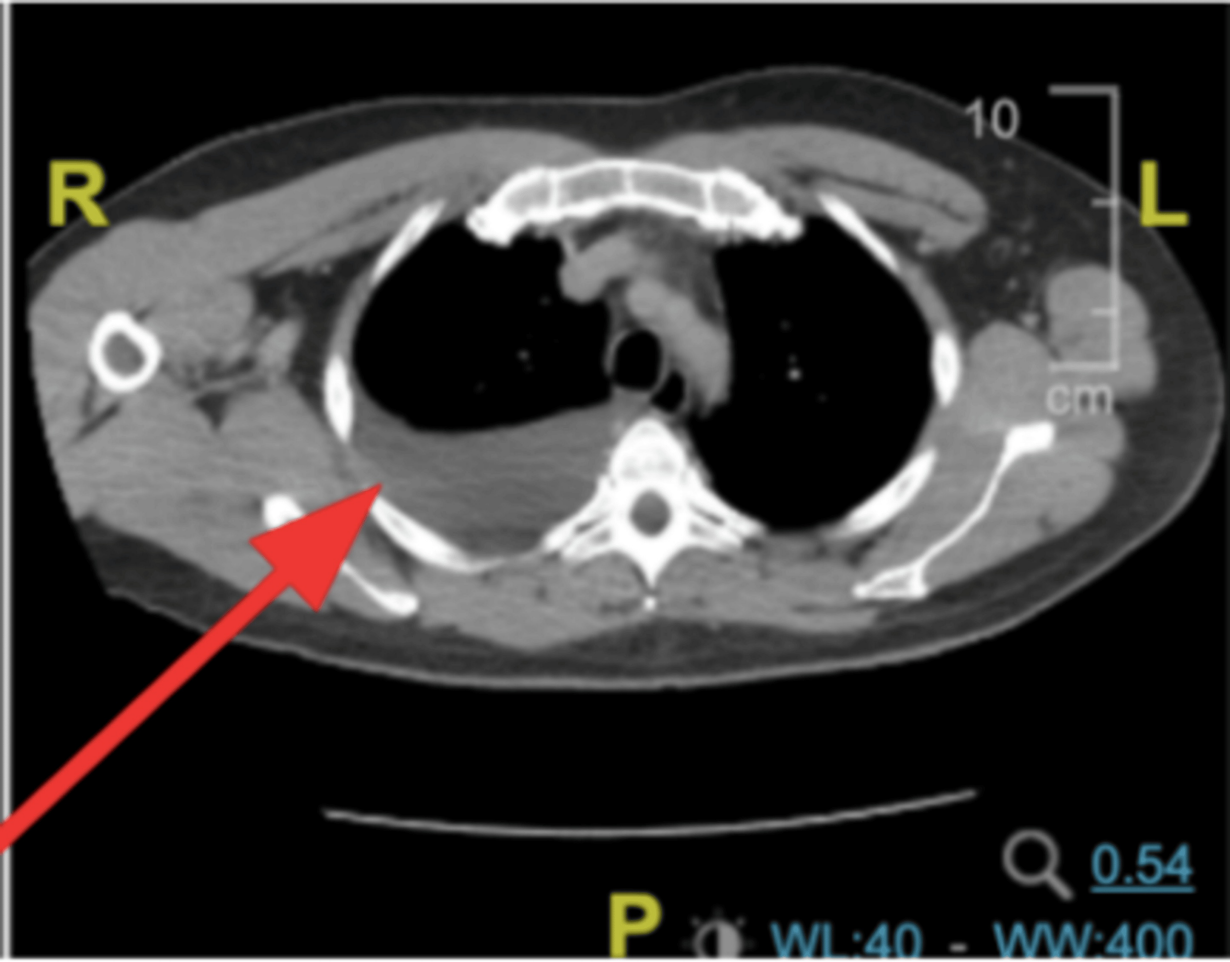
Axial CT of the abdomen with contrast CT scan of the abdomen with contrast showed a consolidation at the right lung base consistent with a large right pleural effusion (red arrow).
